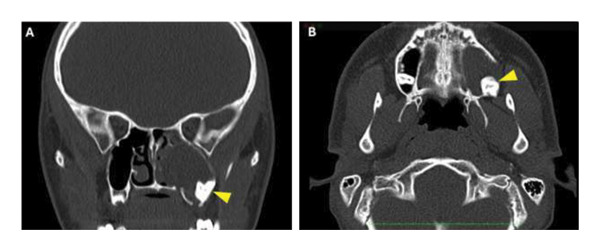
Computed tomography scans of case presentation. (A) Coronal view showing opacification of the left maxillary sinus with bony expansion, left ethmoid opacification, and odontogenic involvement (yellow arrow). (B) Axial view of left maxillary sinus with odontogenic involvement (yellow arrow).
